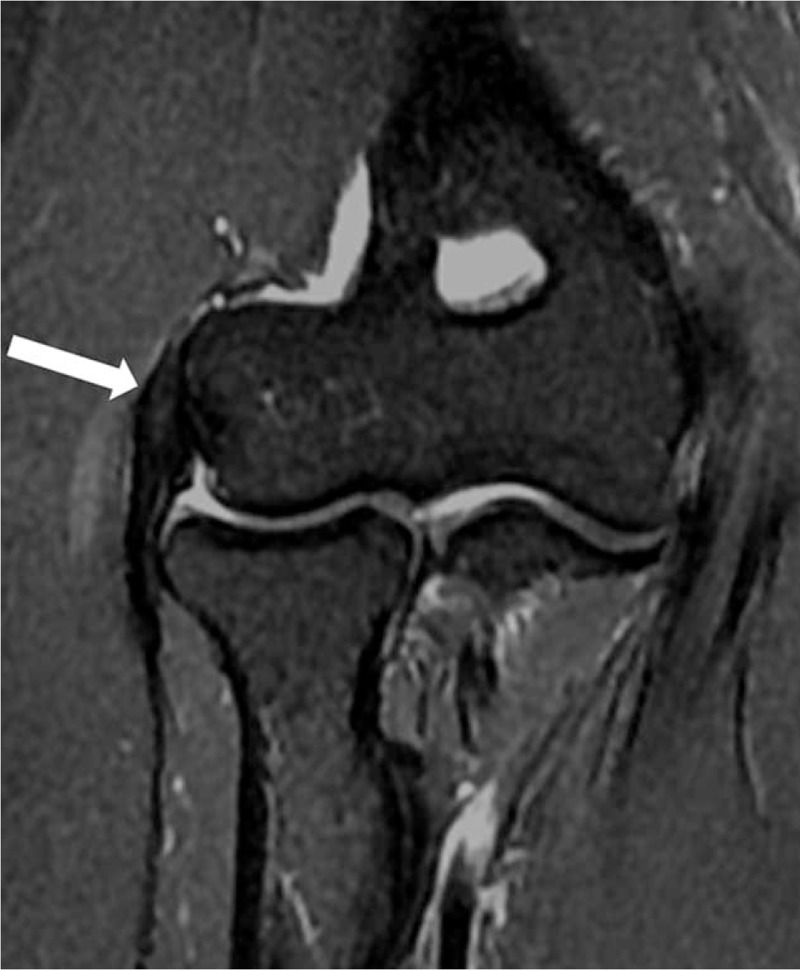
40-year-old man with right elbow pain ∼7 months (Tendinopathy score = 1; PRTEE score = 28). Coronal T2-weighted fat-suppressed MR image shows a mild focal increased tendon signal (white arrow). PRTEE = Patient-Rated Tennis Elbow Evaluation.
